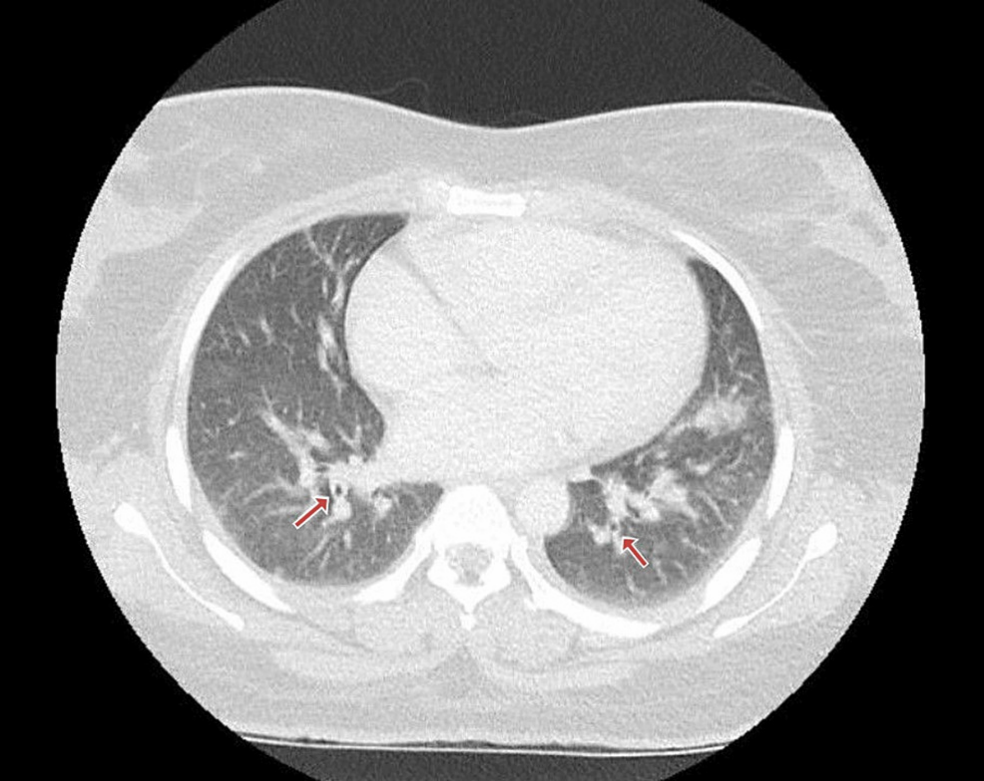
CT of the chest shows multiple cavitary nodules in bilateral lungs
